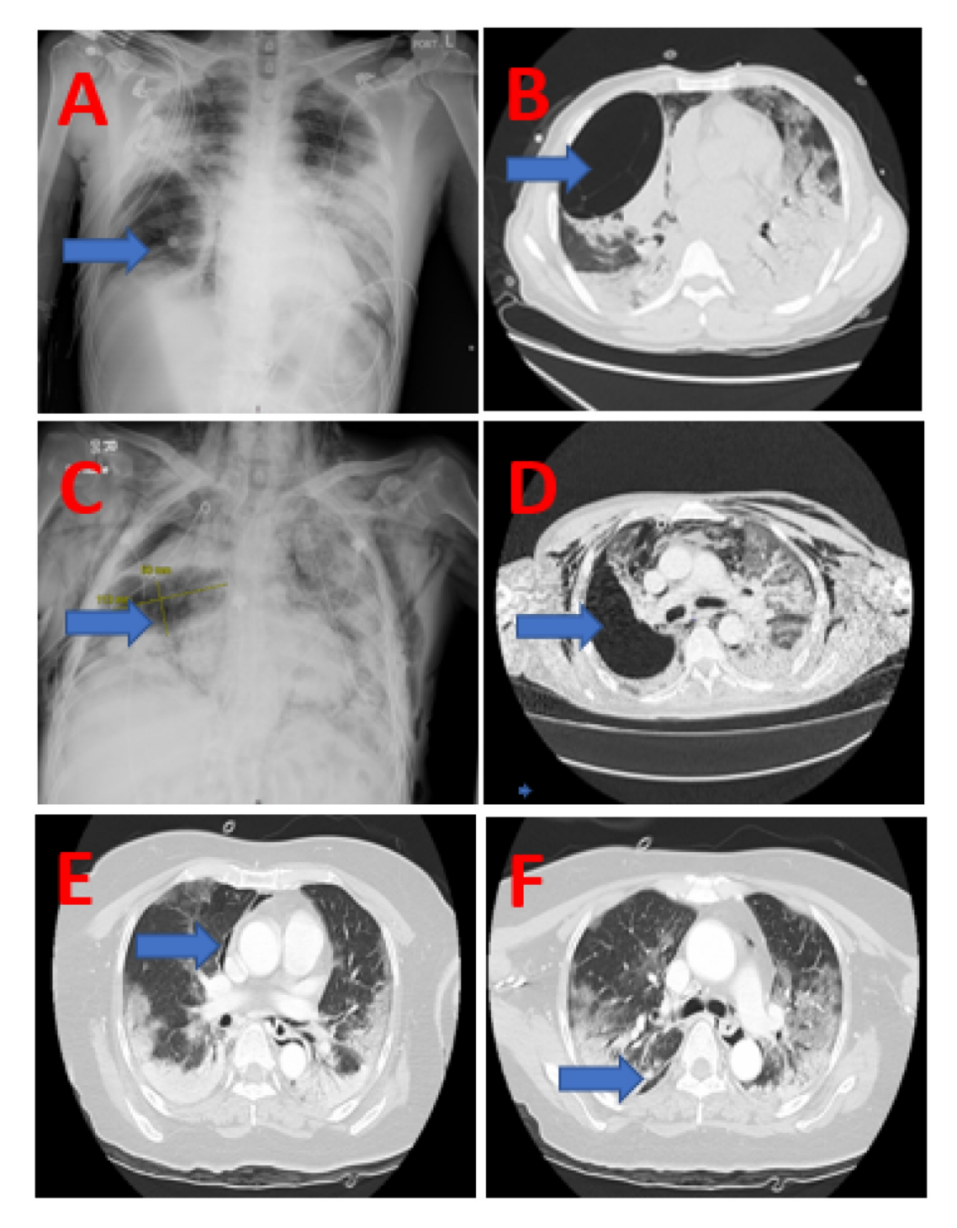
Radiologic findings in patients with COVID-19 and pneumothoraces (A) Case 1 with chest radiograph showing extensive bilateral airspace disease, right pigtail catheter placement and right pneumatocele (arrow). (B) Case 1 with confirmation of findings in (A) on CT scan including pneumatocele (arrow). (C) Case 2 with chest radiograph showing extensive bilateral airspace disease, bilateral chest tube placement and right pneumatocele (arrow). (D) Case 2 with confirmation of findings in (C) on CT scan including right pneumatocele (arrow). (E) Case 3 with CT scan showing evidence of pneumomediastinum along with bilateral air space disease. (F) Case 3 with CT scan showing evidence of small right upper lobe pneumothorax along with bilateral air space disease (arrow).
